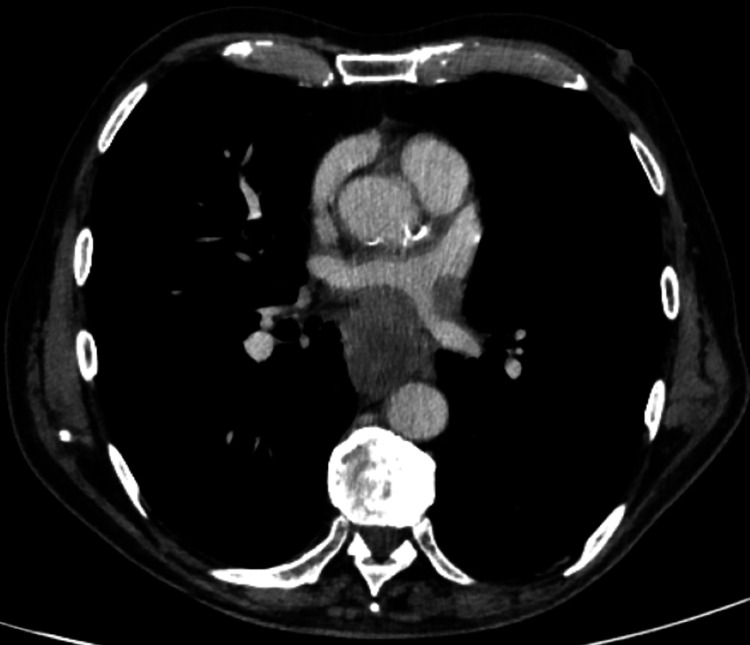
The CTTAP revealing an ill-defined oesophageal mass encasing the left pulmonary vein CTTAP: CT thorax, abdomen, and pelvis
